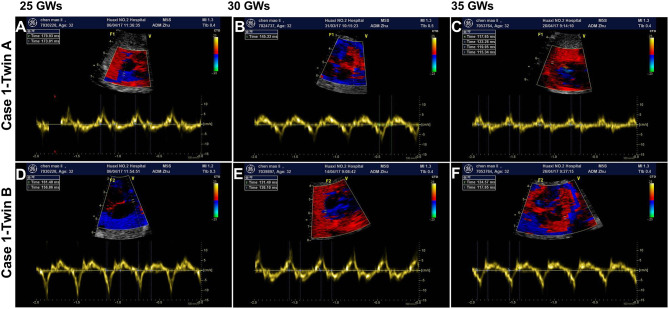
The findings of fetal atrioventricular interval monitored by fetal echocardiography. (A,D) Show the fetal atrioventricular (AV) intervals upon diagnosis (170/160 ms) at 25 gestational weeks (GWs). Panels B/E show the findings (145/136 ms) of medium term after treatment with oral dexamethasone at 30 GWs. (C,F) Show the final echocardiography prior to delivery (130/127 ms) at 35 GWs. (A–C) Belong to the fetus of case 1-twin A, and (D–F) belong to the fetus of case 1-twin B. ms, millisecond.
